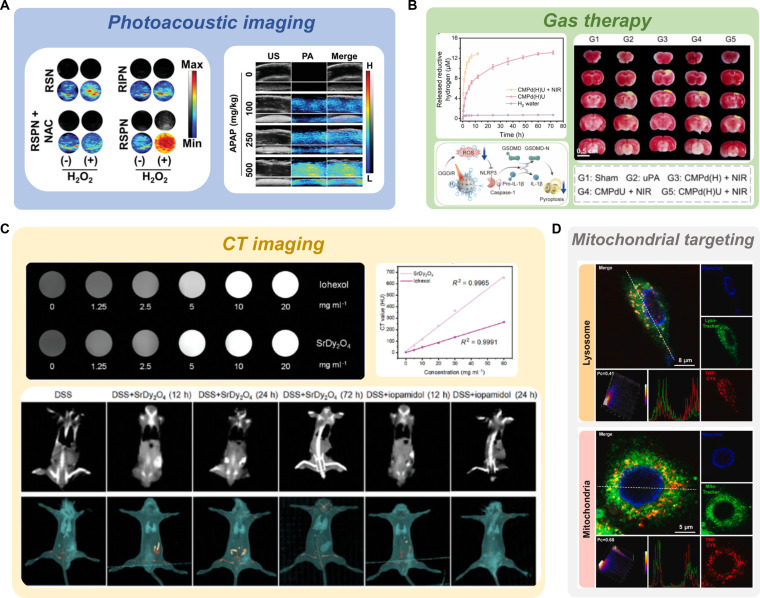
Multifunctional integration of antioxidant nanozymes. (A) ROS-responsive ceria nanozyme probe integrating photoacoustic imaging and ROS scavenging for theranostic application [132]. Copyright 2022, Wiley-VCH. (B) Multifunctional nanozyme platform with targeted drug delivery, sustained ROS scavenging, and neuroprotection for ischemic stroke therapy [133]. Copyright 2025, Elsevier. (C) Acid-stable SrDy_2_O_4_ nanozyme integrating ROS scavenging, intestinal barrier repair, gut microbiota regulation, and x-ray imaging for ulcerative colitis management [118]. Copyright 2025, Wiley-VCH. (D) Microenvironment-responsive metal-phenolic network nanozyme targeting mitochondria to integrate ROS scavenging, pyroptosis inhibition, and extracellular matrix regeneration for intervertebral disc degeneration therapy [134]. Copyright 2022, Elsevier.
